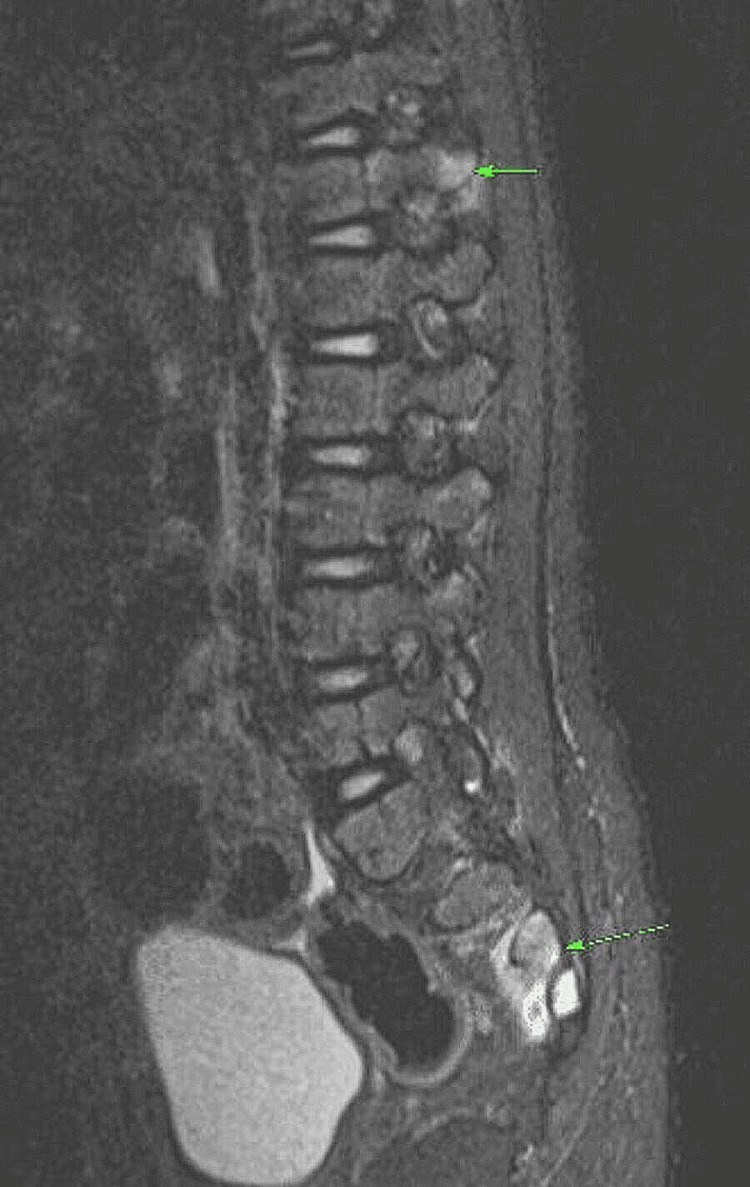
MRI of lumbar spine. Mild bone oedema is seen in right transverse processes of T12, L3, L4, L5, periarticular region of sacrum and lower sacrum bodies (particular adjoining lateral cortices). Areas marked by green arrows are bone oedema.
